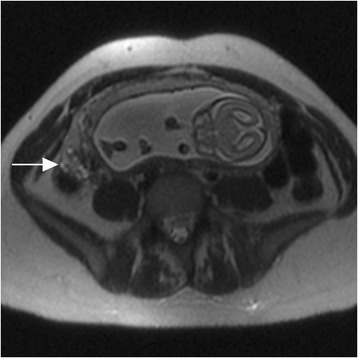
Normal ovary in pregnancy. Axial T2-weighted image with normal right ovary (arrow), demonstrating normal size, ovoid shape, stromal signal intensity, and small follicles
